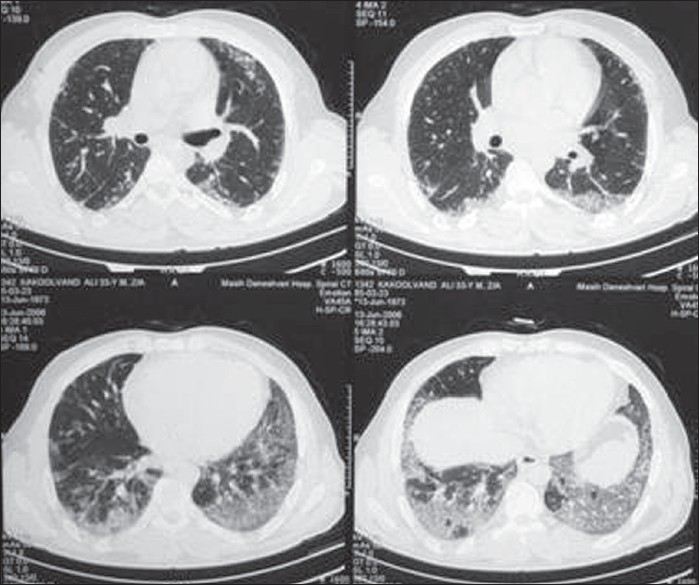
CT scans in nonspecific interstitial pneumonia
